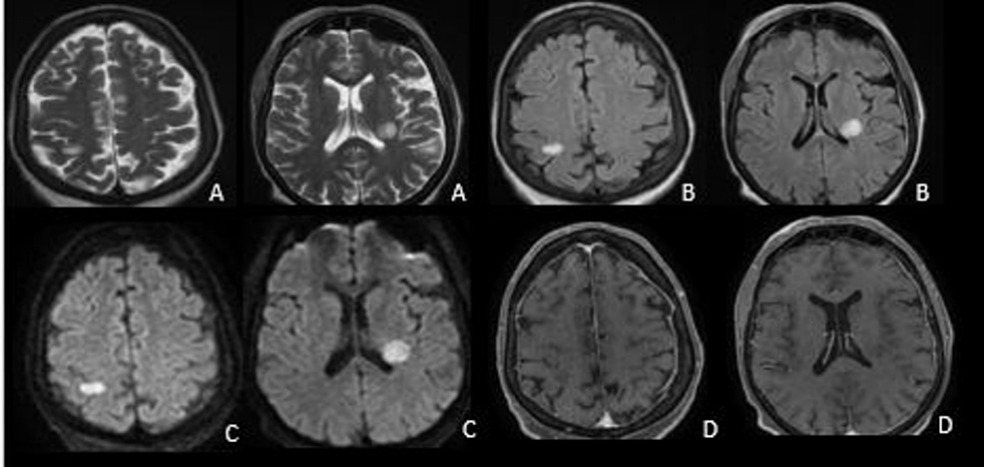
Brain magnetic resonance imaging (MRI) four weeks after admission showing stability of the lesion load with optic atrophy and disappearance of contrast enhancement A) T2 axial view; B) T2 fluid-attenuated inversion recovery (FLAIR) axial view; C) Diffusion axial view; D) T1 Axial view with gadolinium
